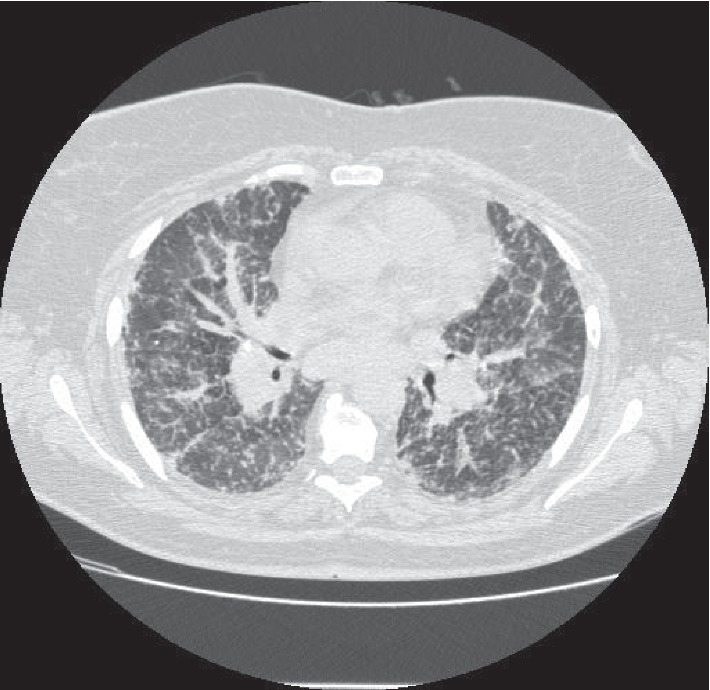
Chest high-resolution computed tomography image showing marked and diffuse alteration of pulmonary parenchyma permeability, thickening of the interlobular septa, and fine bronchovascular and subpleural micronodularity, associated with mediastinal lymphadenopathy.
